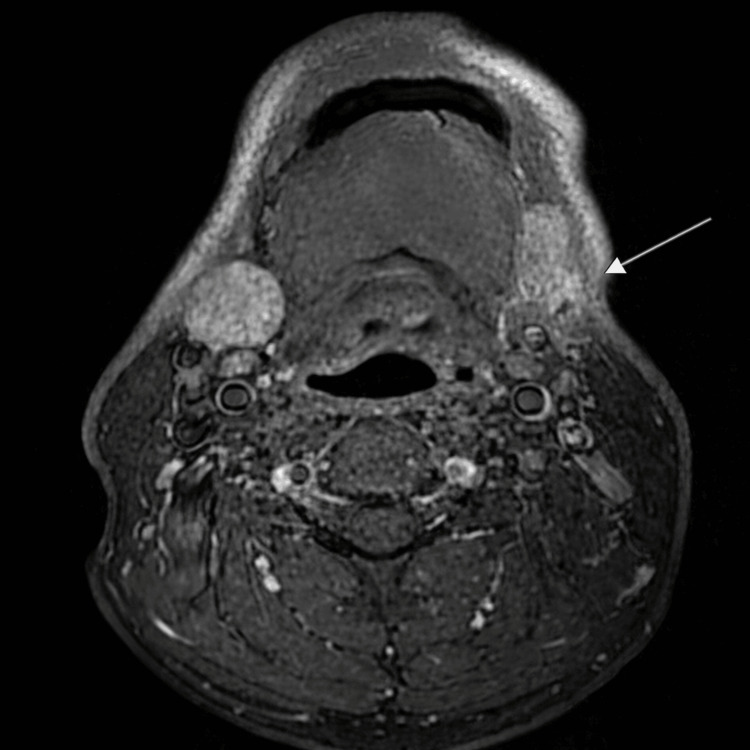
Axial T1 MRI of the neck with contrast showing resection of the left submandibular gland tumor with ill-defined soft tissue infiltration and heterogeneous enhancement in the surgical bed measuring approximately 2.4 x 2.5 x 2.1 cm, involving the cutaneous and subcutaneous region.
